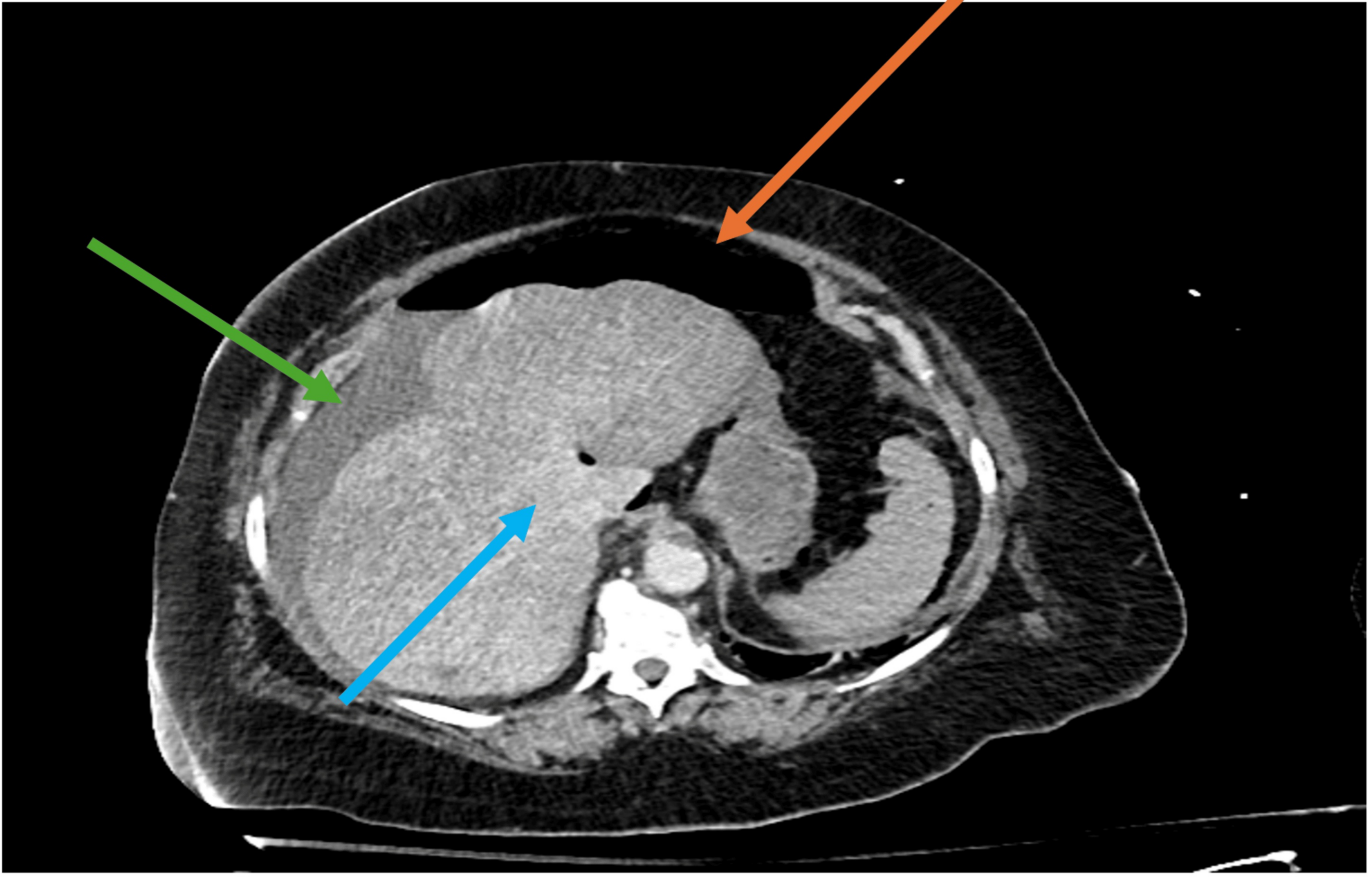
Computed tomography demonstrating liver hypo attenuation. Orange arrow points to pneumoperitoneum. Green arrow points to free fluid. Blue arrow highlights area of liver hypo-attenuation.
